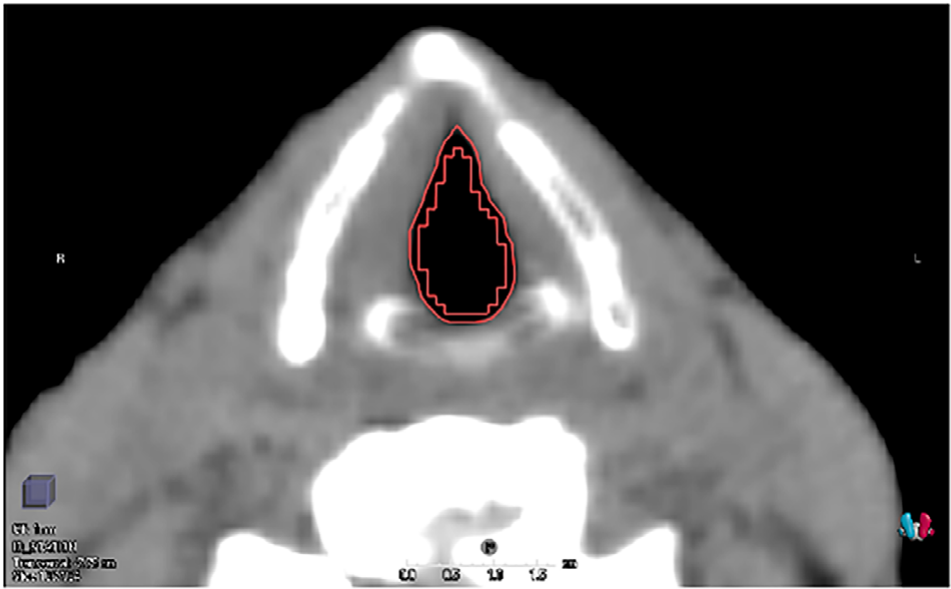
Axial CT images for delineation of surface ROIs. CT, computed tomography; ROI, region of interest.
